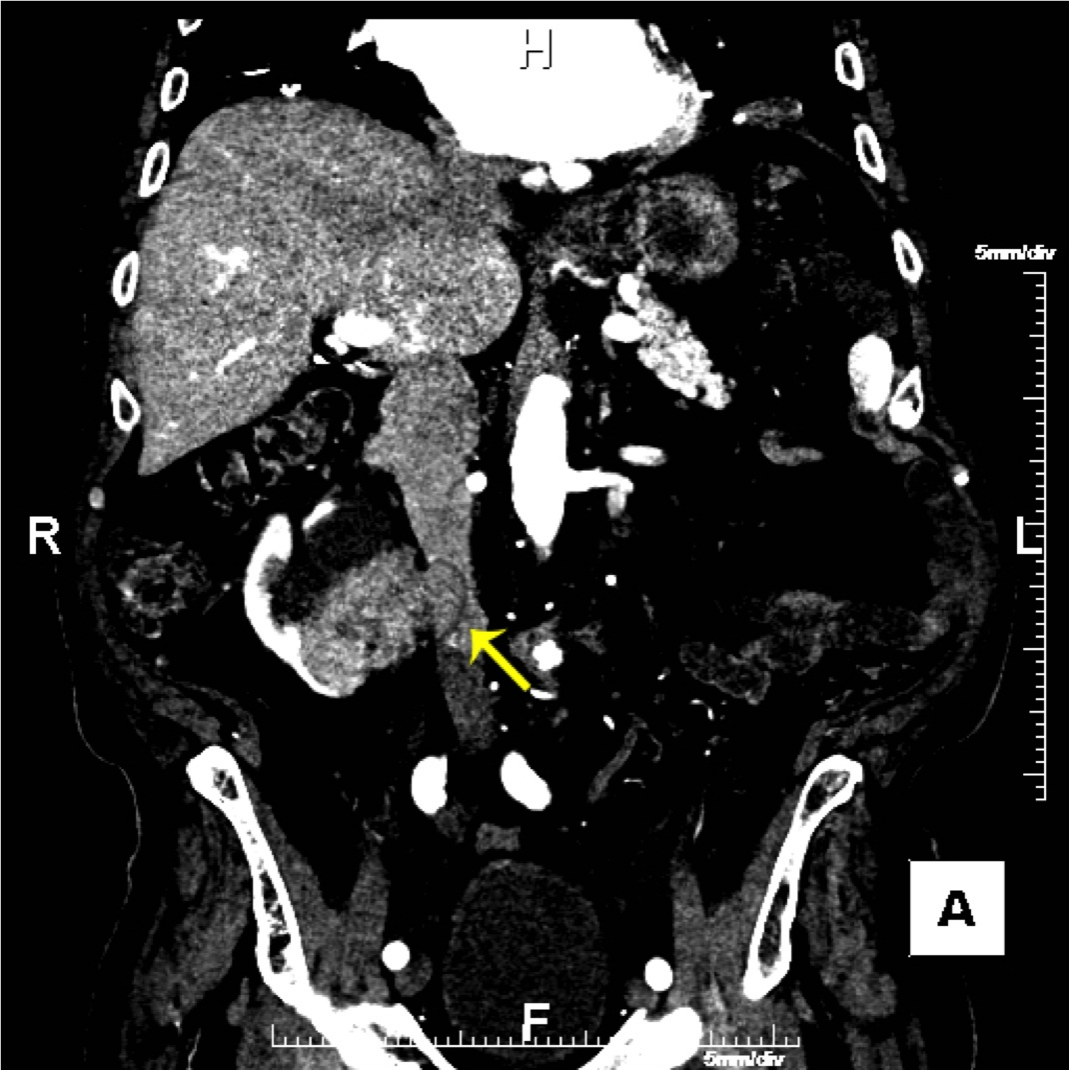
A Contrast-Enhanced CT of the Abdomen and Pelvis Indicating Renal Cell Carcinoma A coronal contrast-enhanced CT of the abdomen and pelvis: renal cell carcinoma of the lower pole of the right kidney with invasion of the accessory right renal vein and inferior vena cava (yellow arrow).
